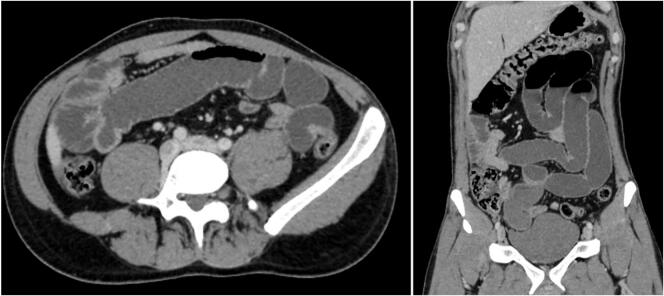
CT scan views suggesting the presence of a tabulated diverticular structure, hanging from the terminal ileum, distended with air-fluid content with a wall that presents a lack of focal enhancement and a focal stricture. The ileal distension described above sits upstream of this structure: the whole evokes a mechanical OIA of the small intestine upstream of a complicated Meckel's diverticulum.
